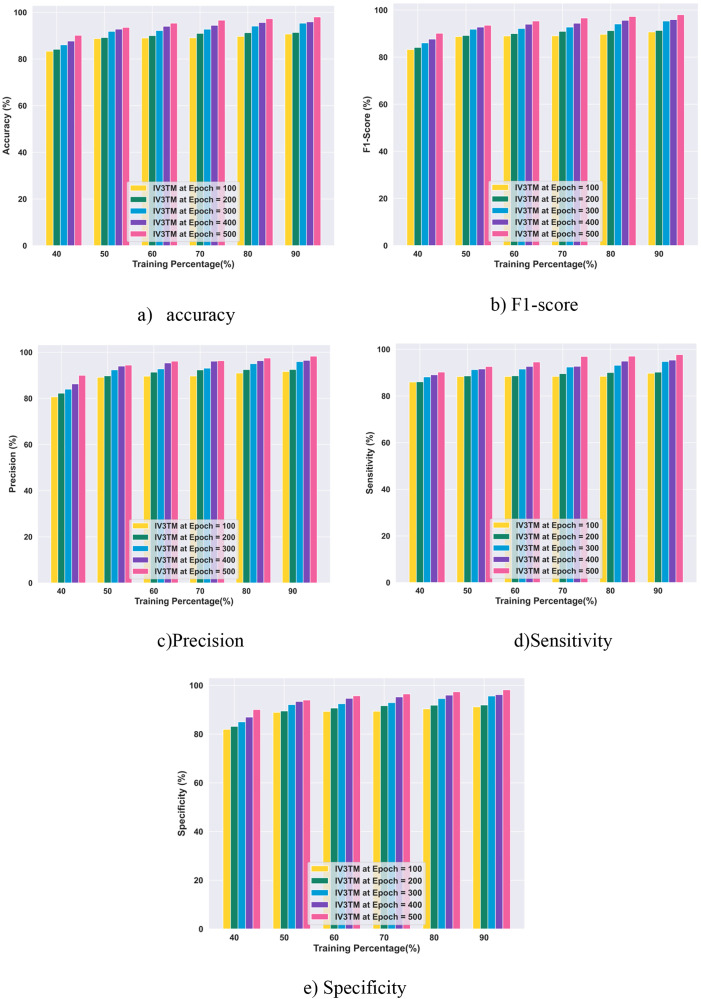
Performance Evaluation with Training Percentage using Brain MRI Dataset.
